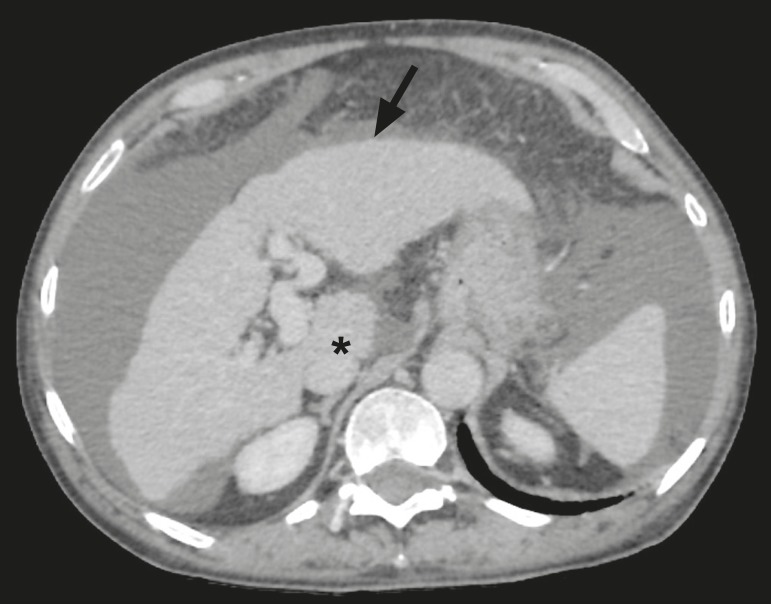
Findings indicative of chronic liver disease. CT in the portal phase, with signs of chronic liver disease: widened fissures, heterogeneity of the hepatic parenchyma, irregular contours, with prominence of lateral segments of the left lobe (arrow) and of the caudate lobe (asterisk), and atrophy of segment IV and of the right lobe. Note also the signs of portal hypertension, including ascites and increased portal vein diameter.
